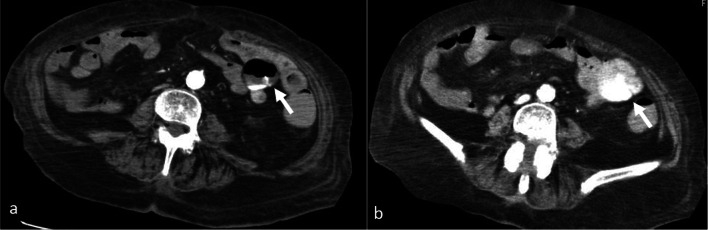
Contrast-enhanced computed tomography of the abdomen. a Early phase. b Delayed phase. Arrow: extravasation around the upper jejunum
